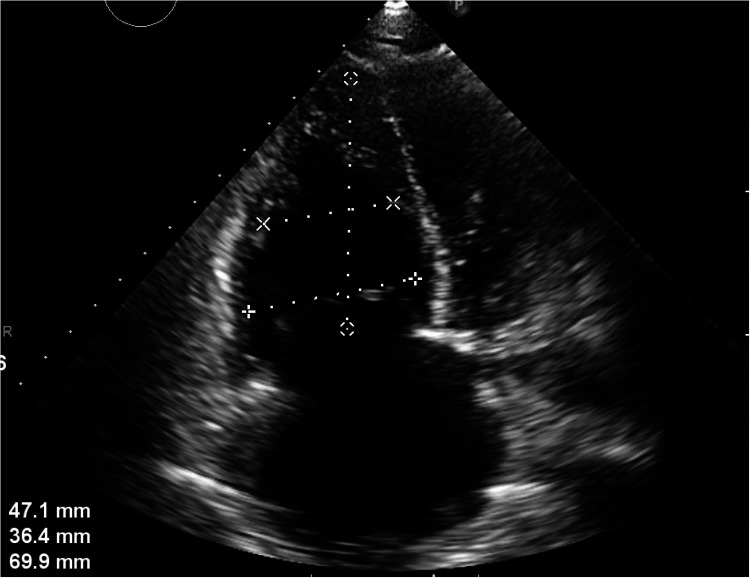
Apical four-chamber section: the right heart is significantly enlarged and compresses the left heart, showing a “D-sign.” The right ventricular end-diastolic diameter is approximately 47.1 × 69.9 mm.
